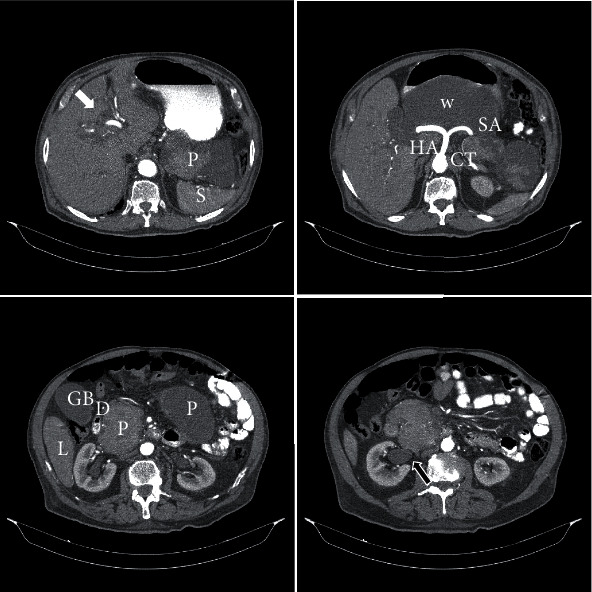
Preoperative CT scan. White arrow: biliary tree dilatation, Black arrow: right hydronephrosis. CT: celiac trunk; D: duodenum; GB: gallbladder; HA: hepatic artery; L: liver; P: pancreas; S: spleen; SA: splenic artery; W: Wirsung duct.
